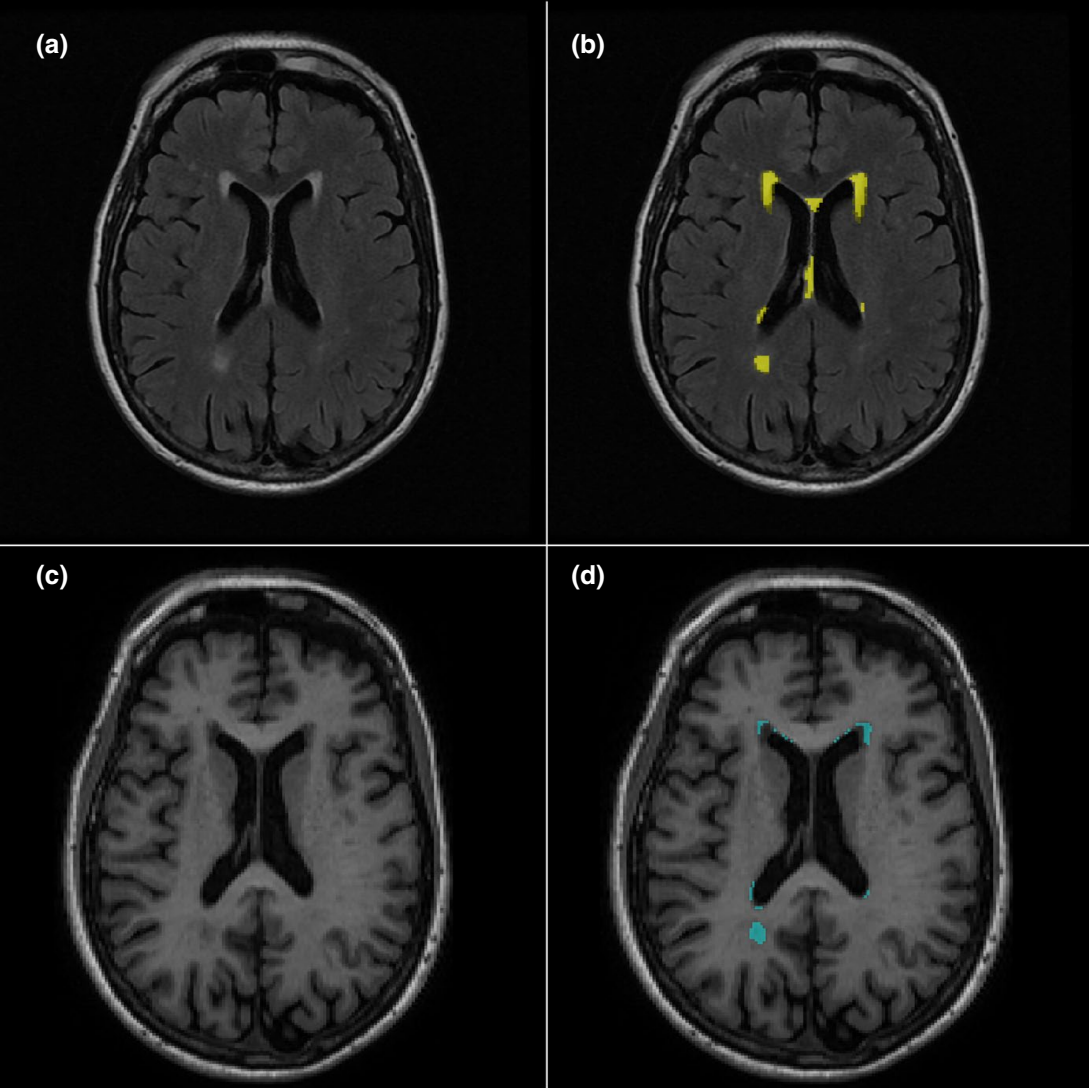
White matter lesions appear bright (WM‐hyper) on T2‐FLAIR (a) and dark (WM‐hypo) on T1‐FSPGR (c) from a representative individual. Automatically identified WM lesions are shown overlaid on anatomic images with a yellow mask for WM‐hyper in (b) and with a blue mask for WM‐hypo in (d)
